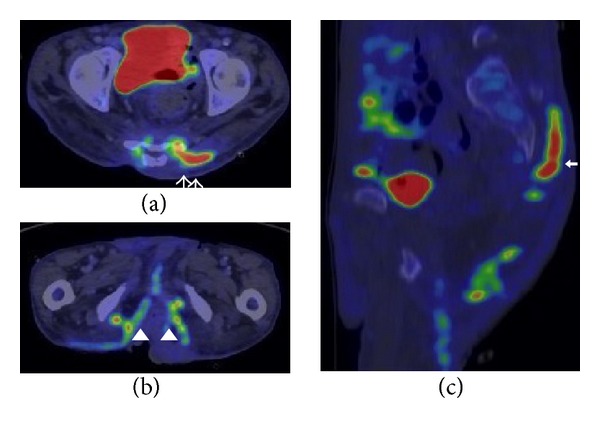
PET-CT performed after surgical excision of the right squamous cell carcinoma in the right thigh with wide resection margins. Note: FDG-avid foci in the perianal spaces ((b), arrowheads) and a more superior abscess collection at the left posterior back ((c), thick arrow), extending to the sacral level ((a), white arrows).
